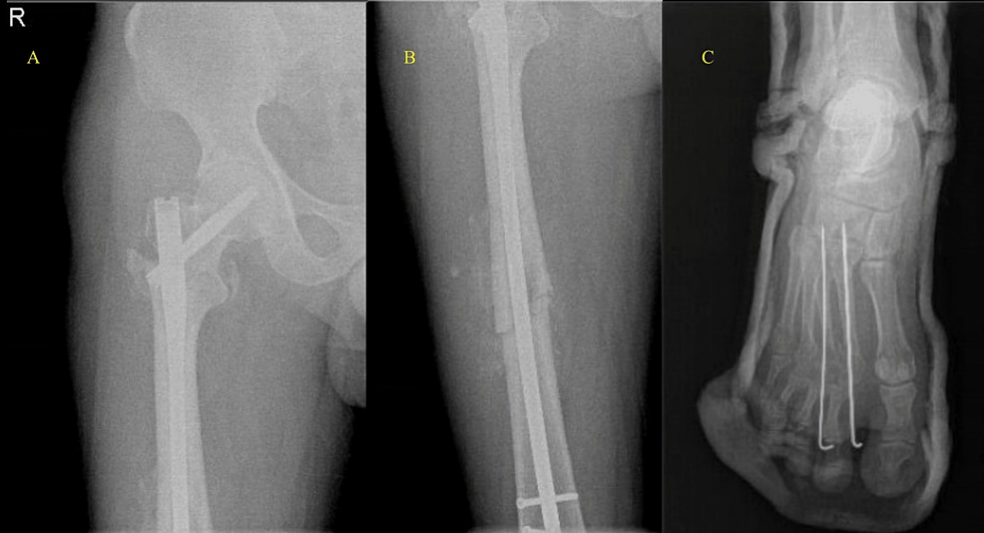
Two weeks postoperative X-rays for the femur fractures (A, B) and metatarsal fractures (C)
